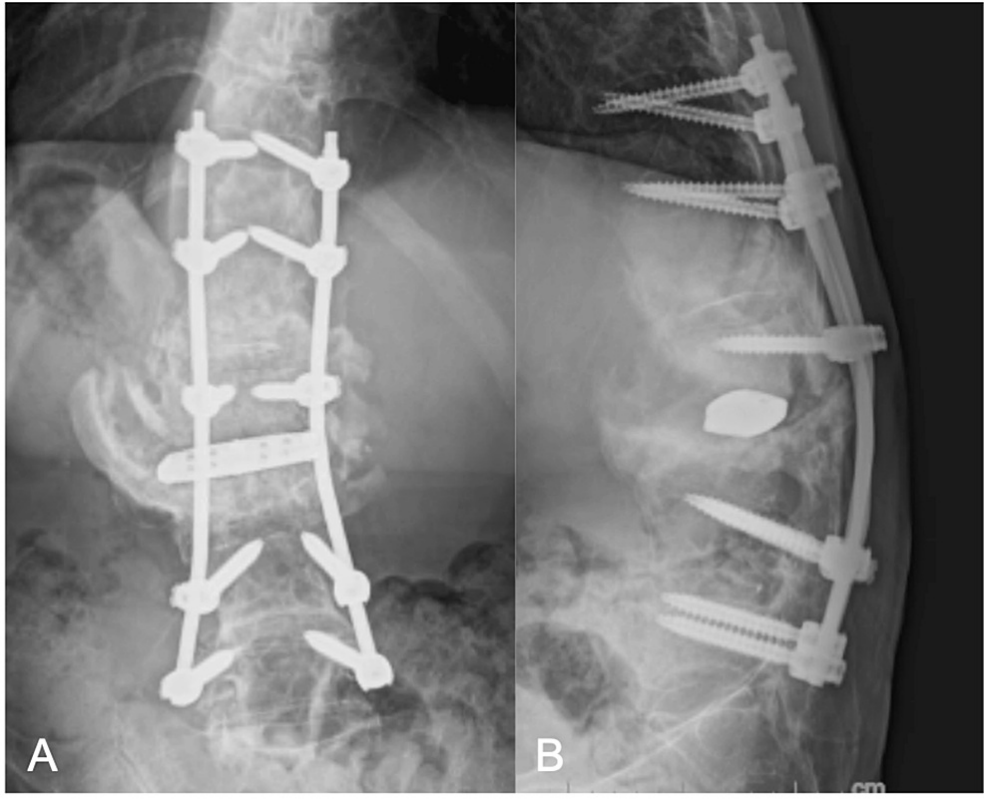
A, B. Postoperative radiograph showing anterior reconstruction with LLIF and posterior spinal fixation with PPS. LLIF: lateral lumbar interbody fusion; PPS: percutaneous pedicle screw
